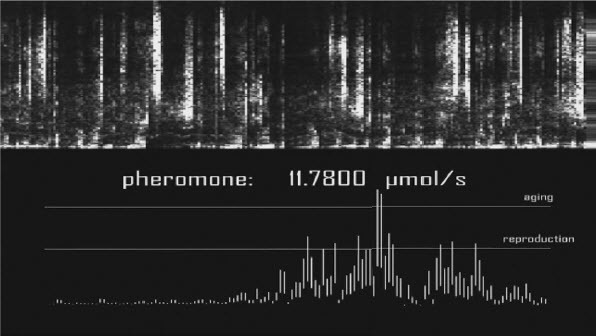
Microbial Keywording*, Ars Electronica Linz, 2019. Spectrogram lead Pheromone Addition and Voice Sonogram, Wall Screen. © *Spiess*
